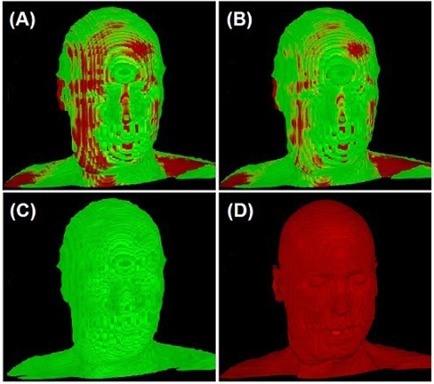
Correction of misalignment in a co‐registered PET/CT image: before (a) and after (b) the 3DVIR correction. The volumetric skin landmark in PET and CT images are shown in (c) and (d), respectively. In PET, the normal tissue uptake of ${\;}^{18}F-\text{FDG}$ can indicate some specific anatomical structures, which were used for registration, as shown in (c). Note that the best volumetric match would show most homogeneity of the color distribution on the skin landmark.
